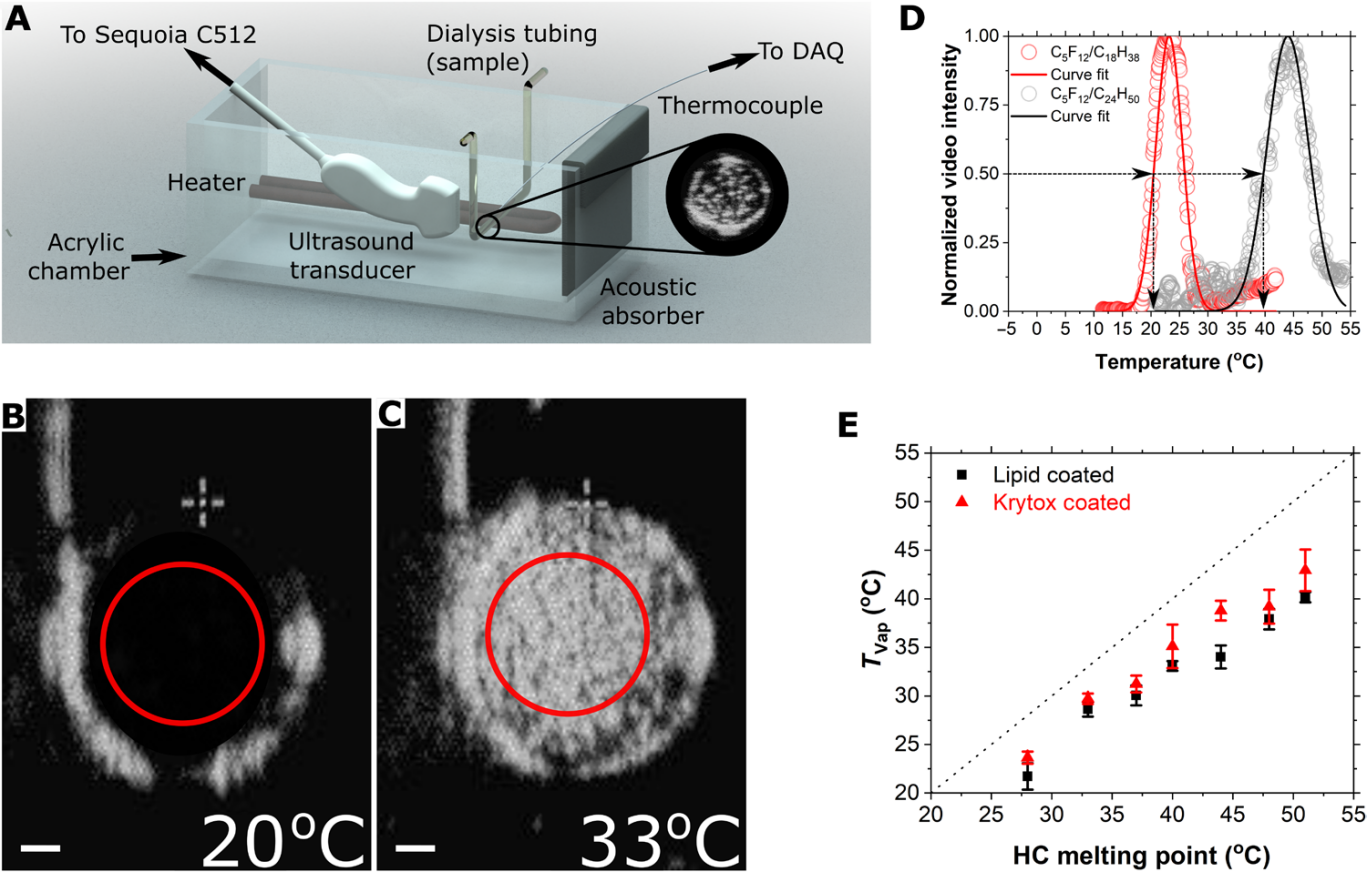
Acoustic verification of the linear dependence of FC droplet vaporization temperature with HC skeleton melting point. (A) Setup used for ultrasound experiments. (B and C) B-mode image of the cross section of a tube filled with endoskeletal droplets at different temperatures. Ultrasound waves are travelling from right to left. Red circles represent the ROI selected for image analysis. The bright vertical line on the top left is the thermocouple. The brighter image in (C) is due to echogenic bubbles formed by droplet vaporization at the higher temperature. Scale bar, 1 mm. (D) Typical video intensity curves for C_5_F_12_/C_18_H_38_ droplets (red) and C_5_F_12_/C_24_H_50_ droplets (black). Solid line represents a Gaussian fit done on the data points. Arrows denote how the vaporization temperature was estimated. (E) Linear dependence of the FC droplet vaporization temperature (mean ± SD) with the HC melting point. Red triangles represent droplets stabilized by a fluorosurfactant (Krytox), and black squares represent droplets stabilized by an HC surfactant (lipids).
